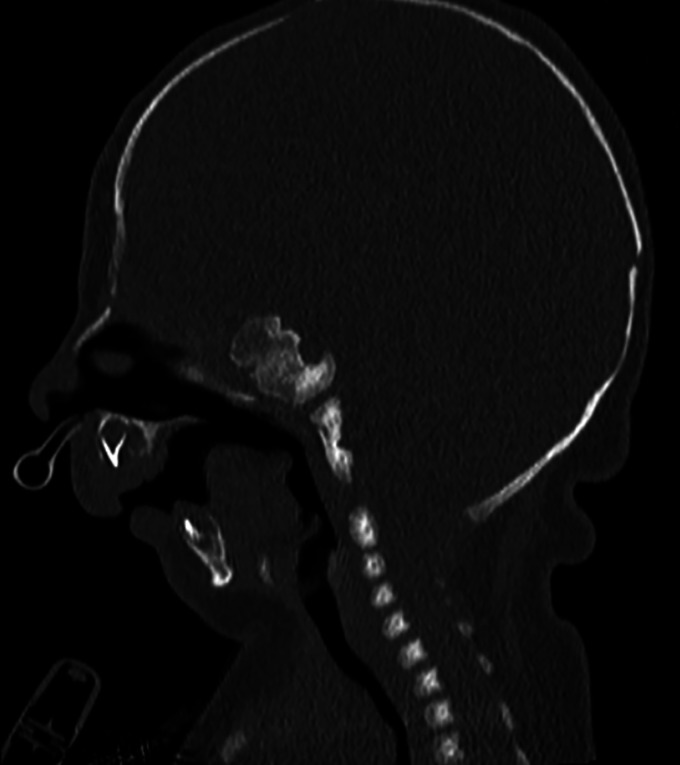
Computed tomography: sagittal view demonstrating severe micro-/retrognathia.
